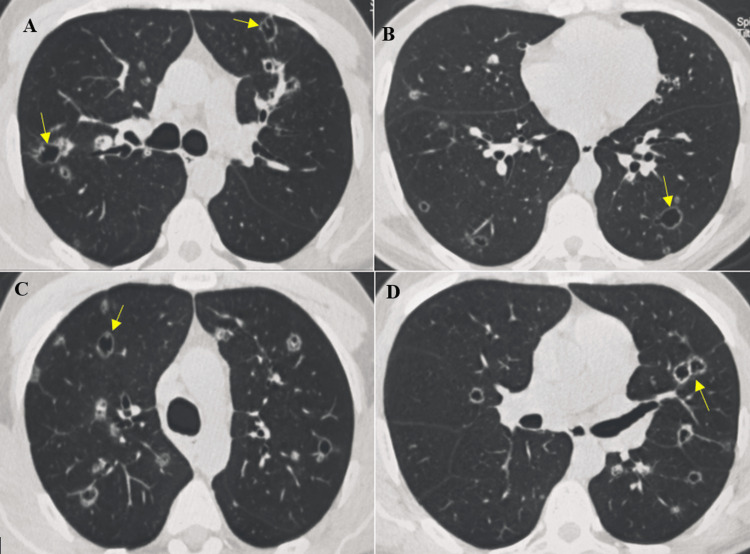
Axial chest computed tomography (CT) images (parenchymal window) A: CT scan showing two pseudocystic lesions in the two upper lobes, right and left (yellow arrow) B: Pseudocystic lesions with a wall < 2mm in the bilateral posterobasal region (yellow arrow) C: Ground-glass nodules in the right subpleural upper lobe with other upper bilateral pseudocystic lesions (yellow arrow) D: Left posterobasal nodule with several ipsilateral pseudocystic lesions and one contralateral lesion (yellow arrow)
